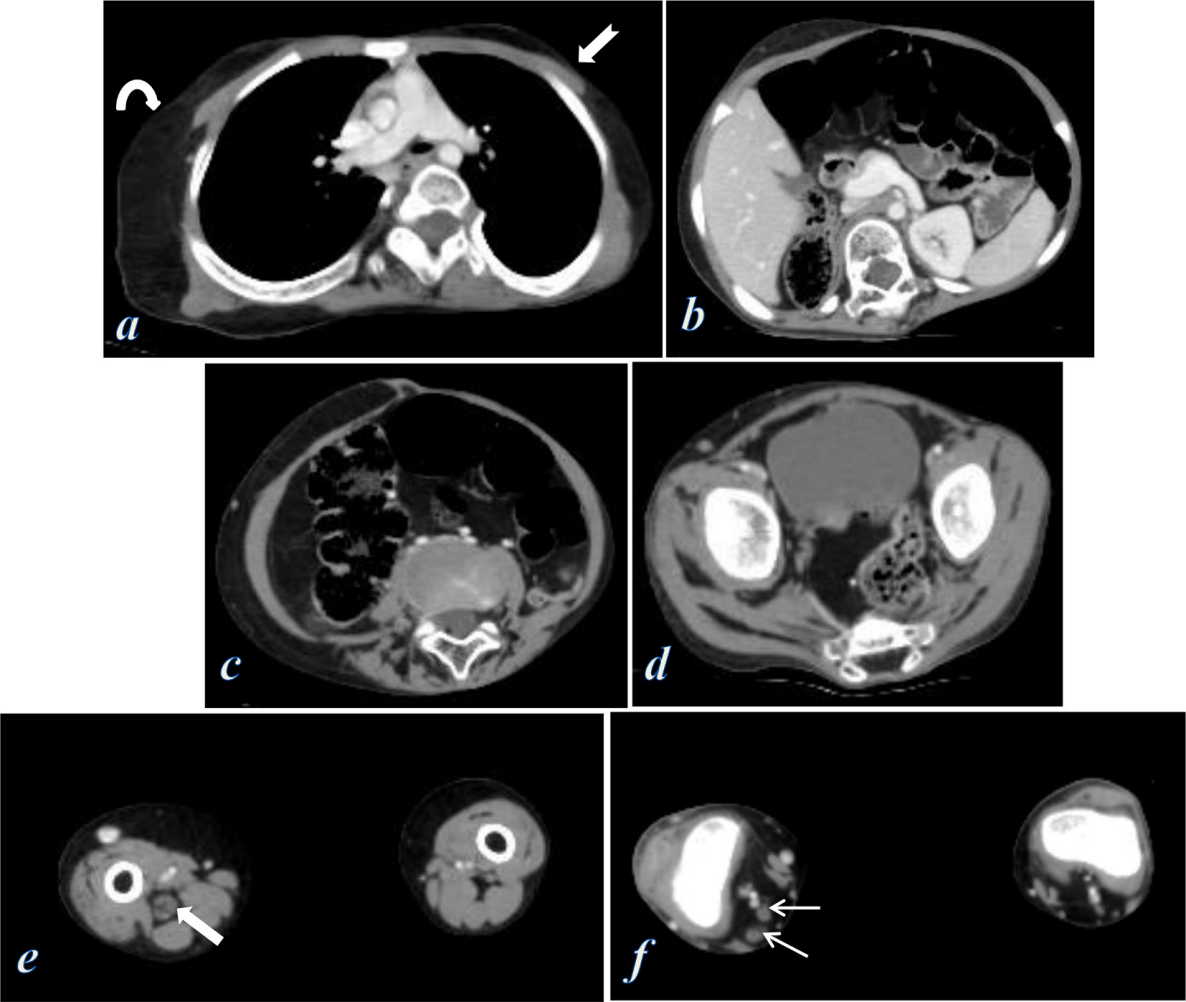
Contrast-enhanced CT scan of the chest, abdomen, pelvis and thighs. (a) Axial image of the chest shows asymmetric subcutaneous adipose fat of the chest wall with areas of increased fat deposition (curved arrow) and other areas of absent subcutaneous fat (notched arrow). (b) Axial image of the upper abdomen shows venous ectasia of the portal, splenic and superior mesenteric veins. (c, d) Axial images of the lower abdomen show irregular asymmetric intra-abdominal and pelvic lipomatosis (evident on the right paracolic gutter and right pararectal region), and irregular asymmetric subcutaneous fat deposition of the abdominal wall with fatty infiltration of the gluteal and erector spinae muscles, also note the venous ectasia of the superficial veins of the abdominal wall. (e, f) Axial images of both thighs show asymmetrically enlarged right thigh with muscular and subcutaneous fat hypertrophy, venous ectasia of the great and short saphenous veins, and diffuse enlargement of the right sciatic nerve (thick arrow), tibial and peroneal nerves (thin arrows) showing interfascicular fat density with no post-contrast enhancement.
